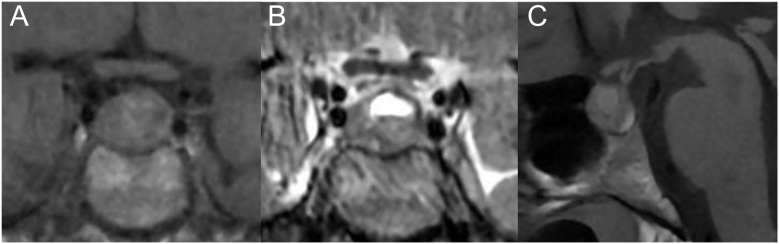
Pituitary MRI performed without contrast injection at 12 weeks of pregnancy showed overall stability of the adenoma. (A) Coronal T1-weighted image. (B) Coronal T2-weighted image. (C) Sagittal T1-weighted image.
